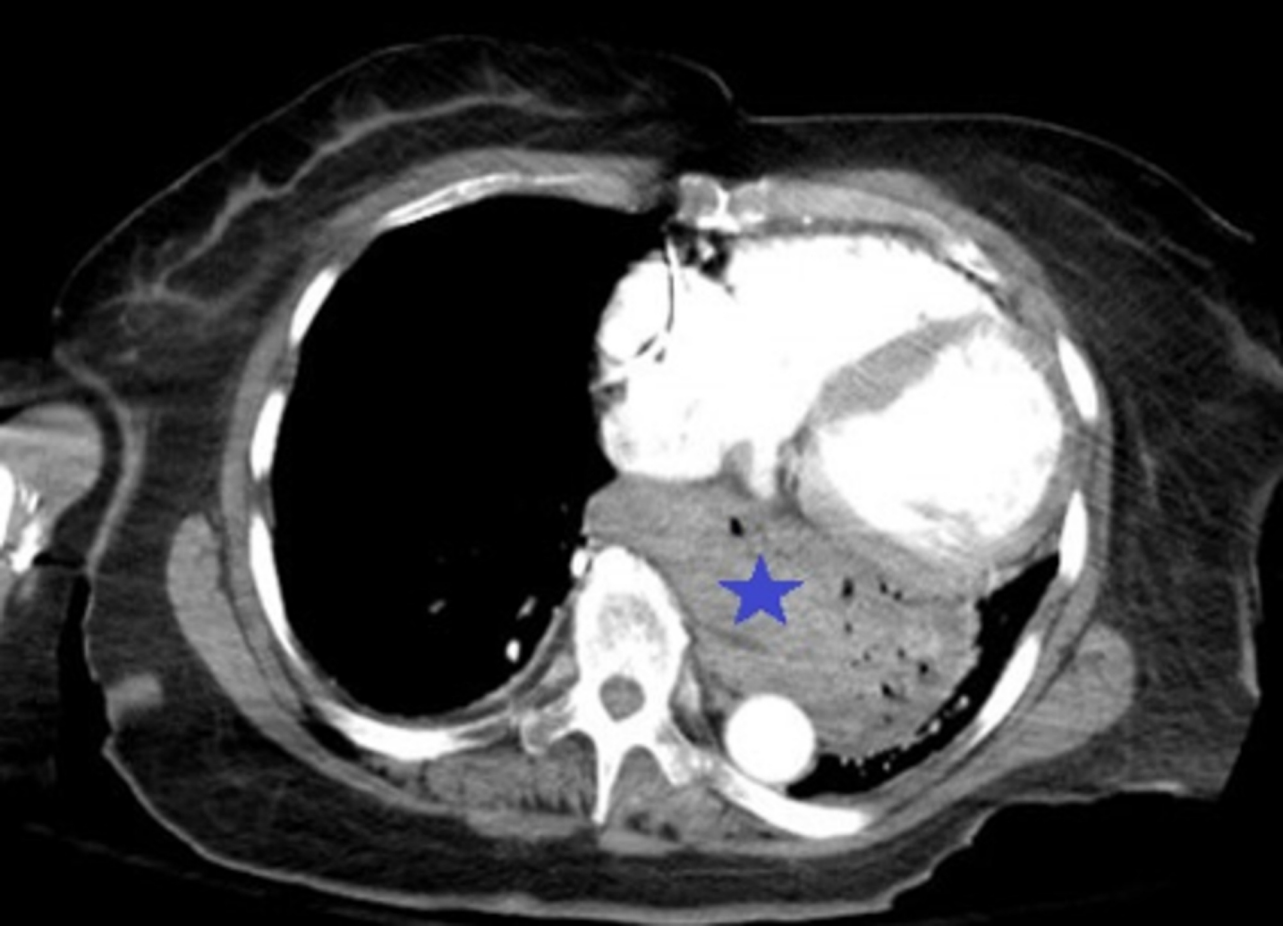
CT image reveals the findings An axial view CT scan of the chest showing the presence of a hiatal hernia (as indicated by the blue star).
